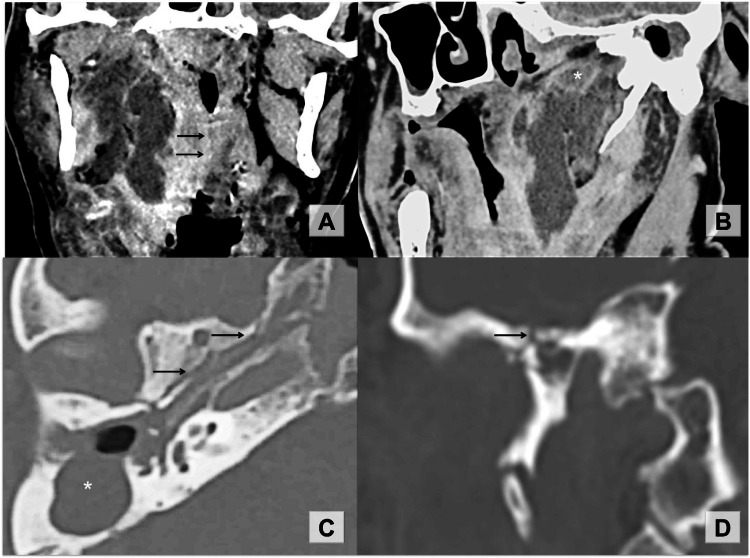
Temporal bone CT, contrast-enhanced scans (A) a multilocular parapharyngeal abscess appears as a lesion with a hypodense center and contrast-enhanced ring on the periphery. Edema and bulging of the pharyngeal wall are shown with the arrows. (B) Oblique sagittal reformat showing infiltration of the deep soft tissues of the skull base (asterisk), peritubal infection spreading route from the middle ear. (C) Oblique axial reformat along the whole peritubal fracture line (arrows). Large cholesteatoma destructed the posterior canal wall and filled the antrum as shown by an asterisk. (D) Comminuted fracture of the petrous paratubal part of the temporal bone (arrow), coronal CT scan.
